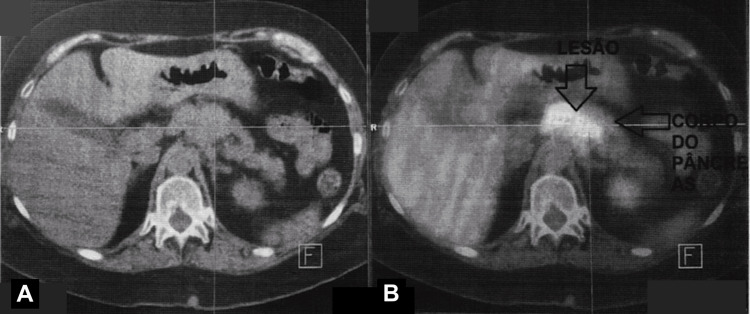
Axial imaging of the gastroesophageal junction A. Axial CT image: contrast-enhanced scan showing a heterogeneous soft-tissue mass at the gastroesophageal junction B. Fused PET/CT image: metabolic fusion image highlighting the hypermetabolic lesion (upper arrow, "LESÃO") and its proximity to the pancreatic body (lower arrow, "CORPO DO PÂNCREAS"), demonstrating loss of distinct fat planes
